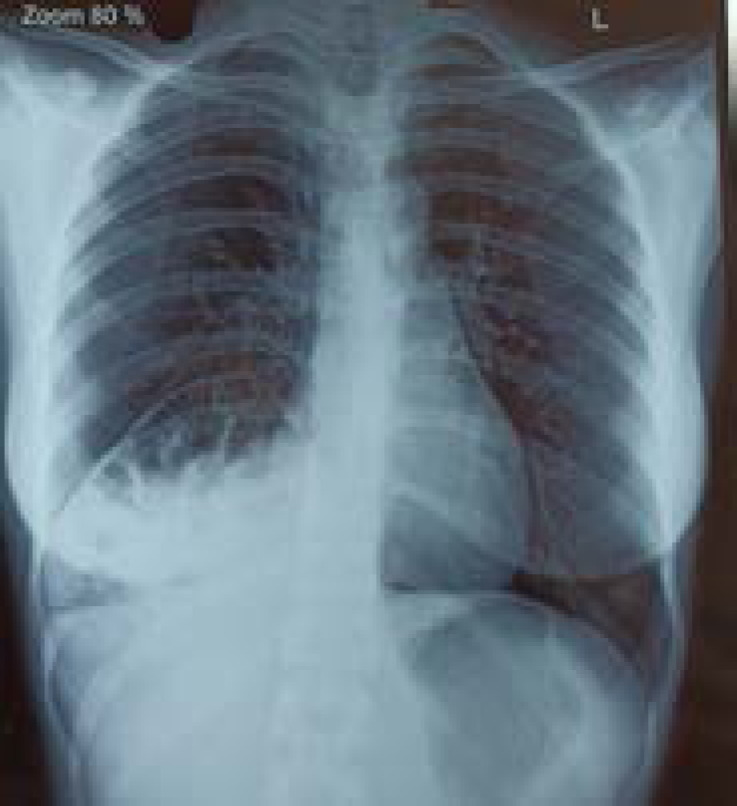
Chest x-ray showing eventration of diaphragm and bowel gases in the right thoracic region.
